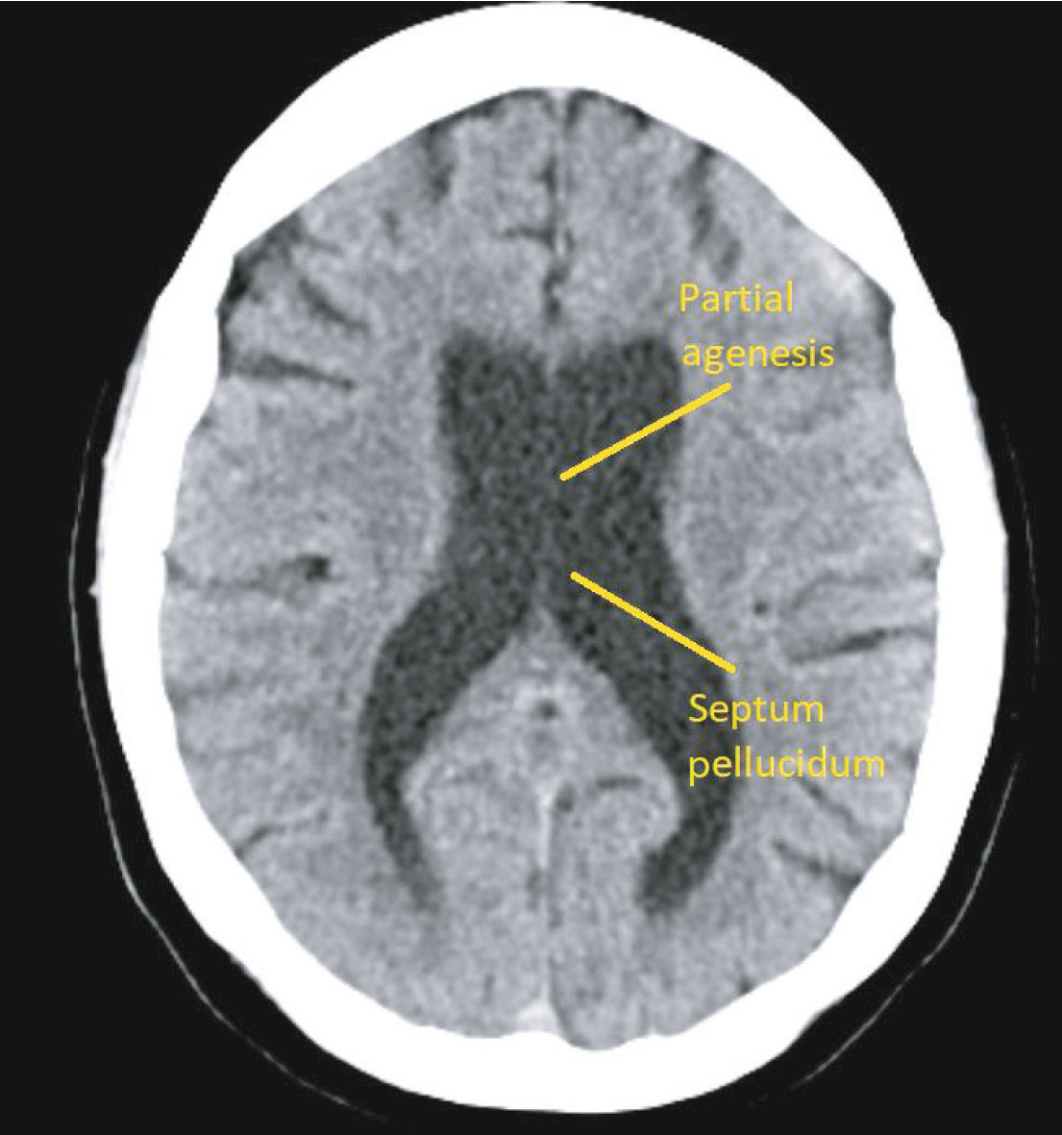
Axial MRI showing septum pellucidum agenesis.
